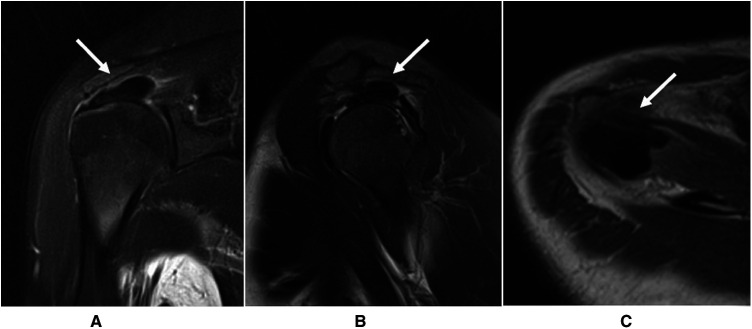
MRI showed the calcific deposits in the target shoulder in in the (A) oblique coronal; (B) sagittal; and (C) horizontal plane.
